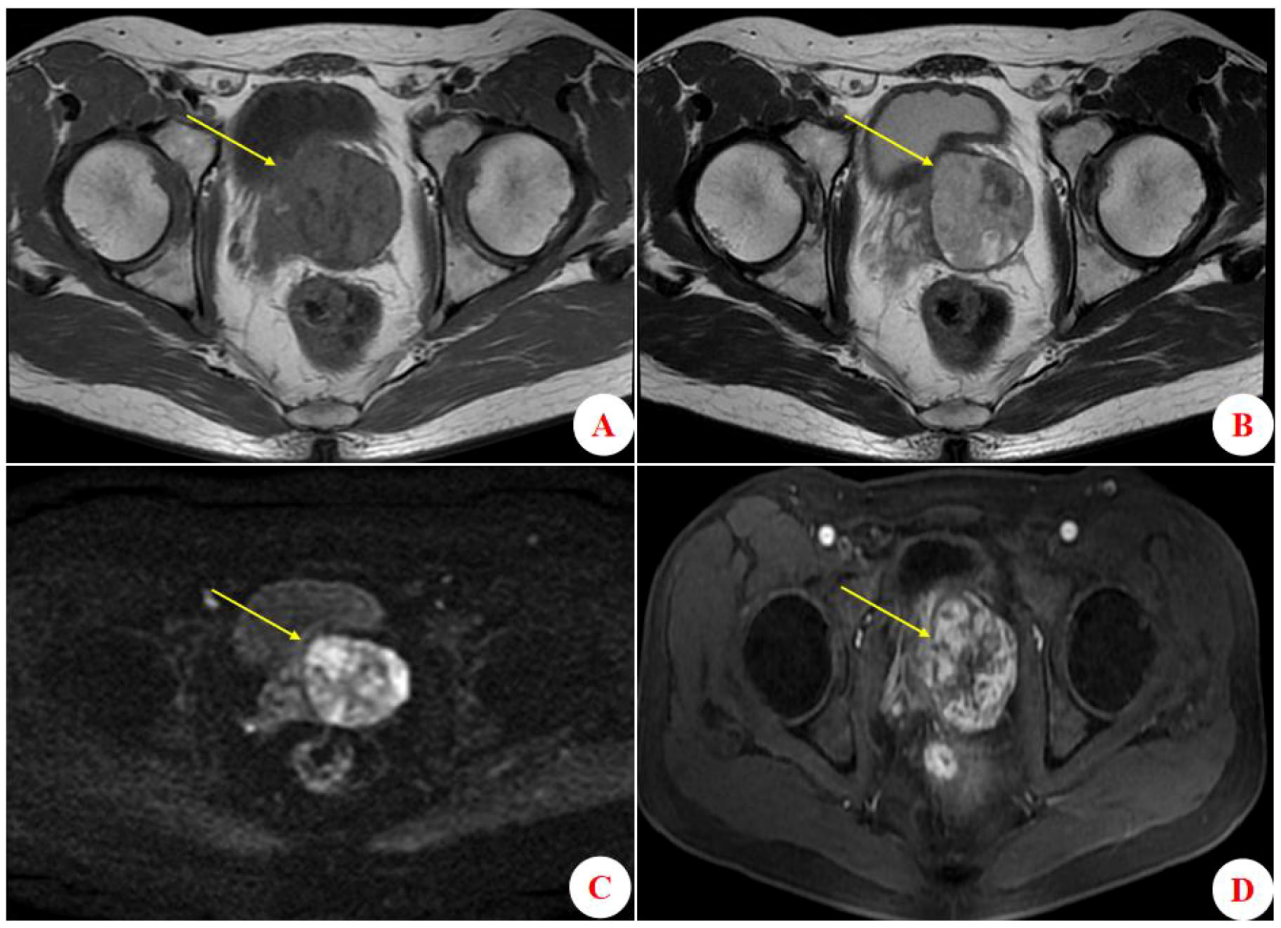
Results of prostate MRI examination. (A) Axial T1-weighted imaging (T1WI) showing a hypointense mass lesion (approximately 5.20 × 5.01 × 4.64 cm) in the left prostate. (B) Axial T2-weighted imaging (T2WI) shows that the lesion is hyperintense. (C) Diffusion-weighted imaging (DWI) reveals diffusional restriction throughout the lesion. (D) Axial contrast-enhanced T2WI shows obvious and persistent enhancement of the lesion, without evidence of marginal invasion.
